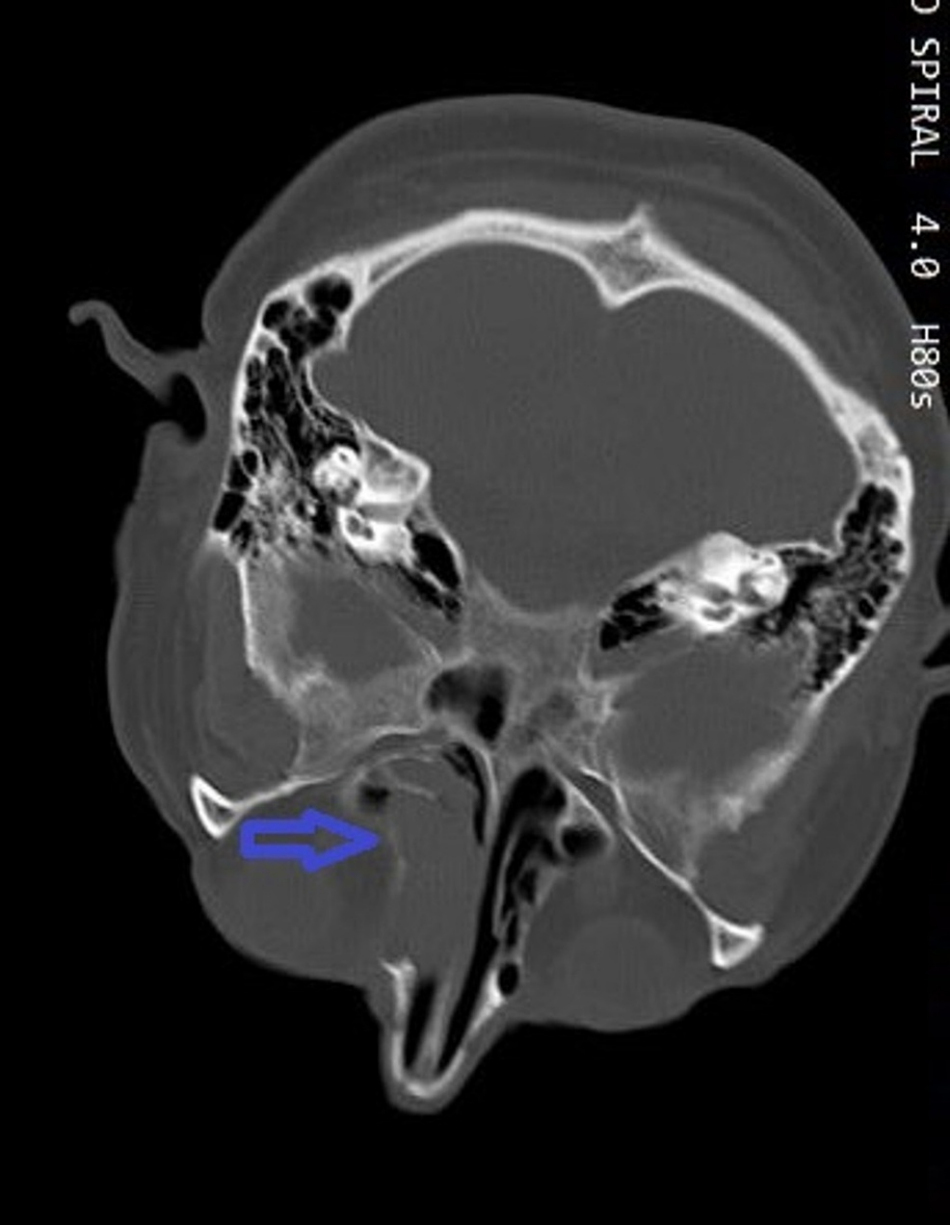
A CT scan (axial view) showing a heterogenous soft tissue mass filling the left nasal cavity (blue arrow) obstructing the choana.
